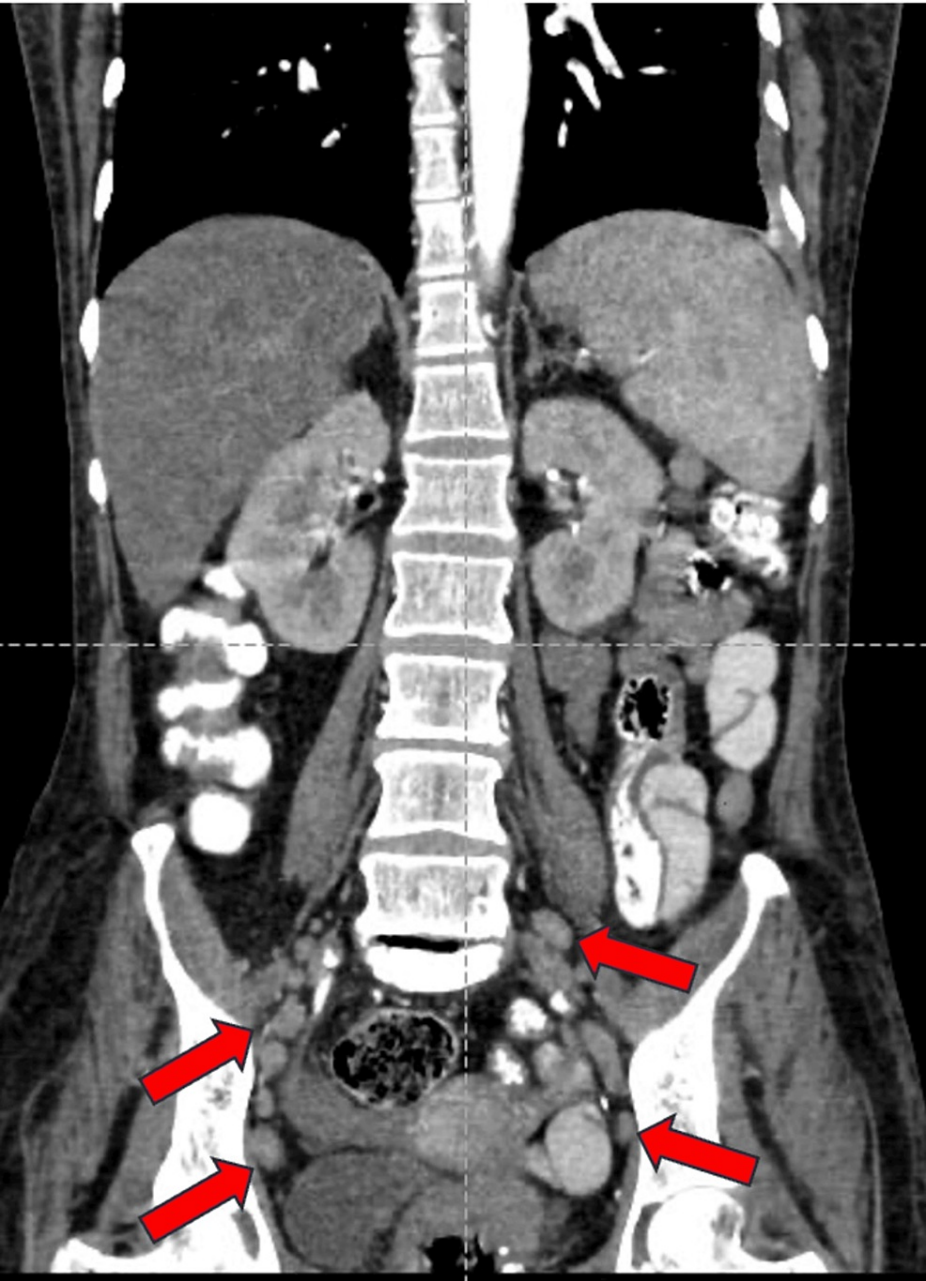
CECT-AP reformatted coronal section showing enhancing lymph nodes along bilateral iliac vessels shown by the red arrows. CECT-AP: contrast-enhanced computed tomography of the abdomen and pelvis
